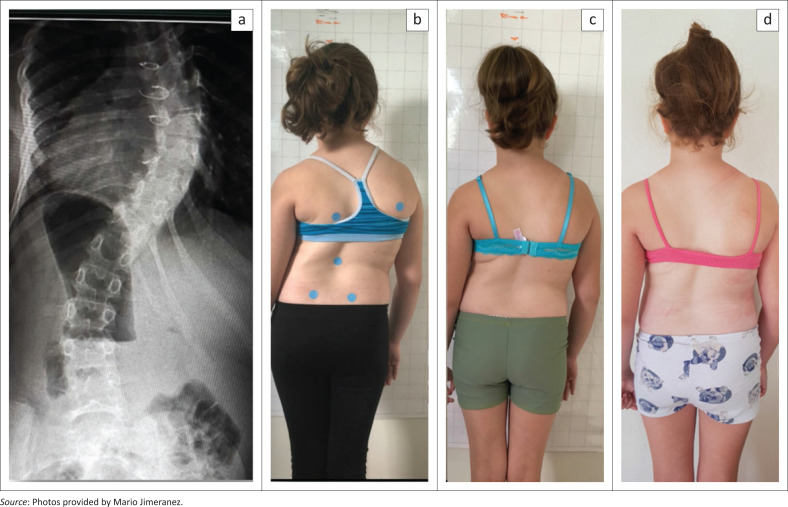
The patient with two operations for congenital heart disease with a collapsing spine at the age of 9.9 years at the start of treatment. (a) X-ray taken on November 2019 with a Cobb angle of approximately 65° Cob, (b) picture from the same time showing the patient from the rear with a collapsing spine, (c) after the application of the Schroth Best Practice Programme whilst waiting for the brace and (d) after a few nights spent in the brace.
